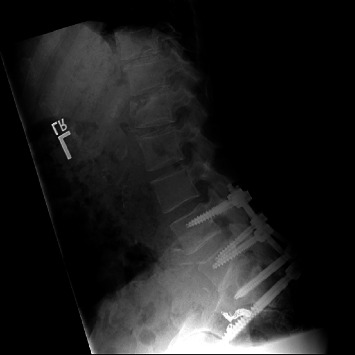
Postoperative X-ray in a patient who underwent percutaneous lumbopelvic fixation.
